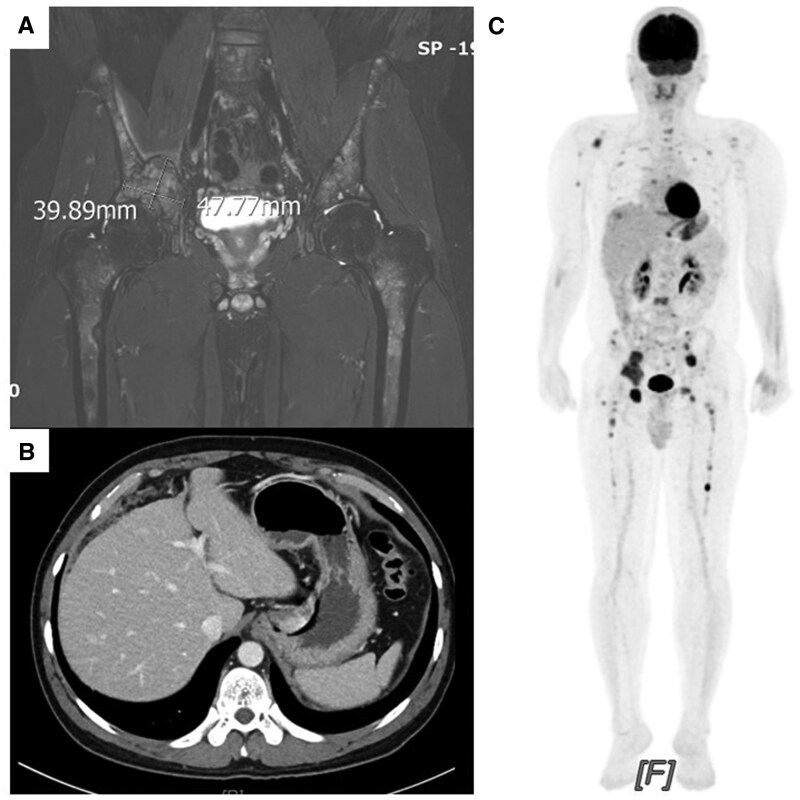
The imaging in the present case of ALK-positive histiocytosis. (A) MR image of the hip is shown Multiple T2 hyperintensity nodular lesions with enhancement of contrast at the covered lower L-spine, bilateral pelvic bones, and bilateral proximal femurs. (B) Contrast-enhanced CT scan of the abdomen revealed reticulonodular infiltration in the omentum and peritoneal hypertrophy, indicating peritoneal seeding. (C) PET/CT scan demonstrated multiple hypermetabolic lesions in stomach, perihepatic soft tissue, mesenteric nodule, and bones.
